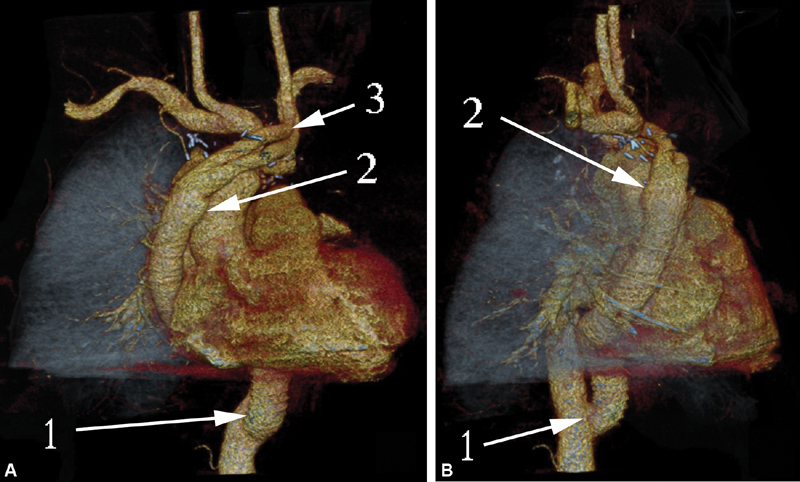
Computed tomography volume rendering frontal view (
